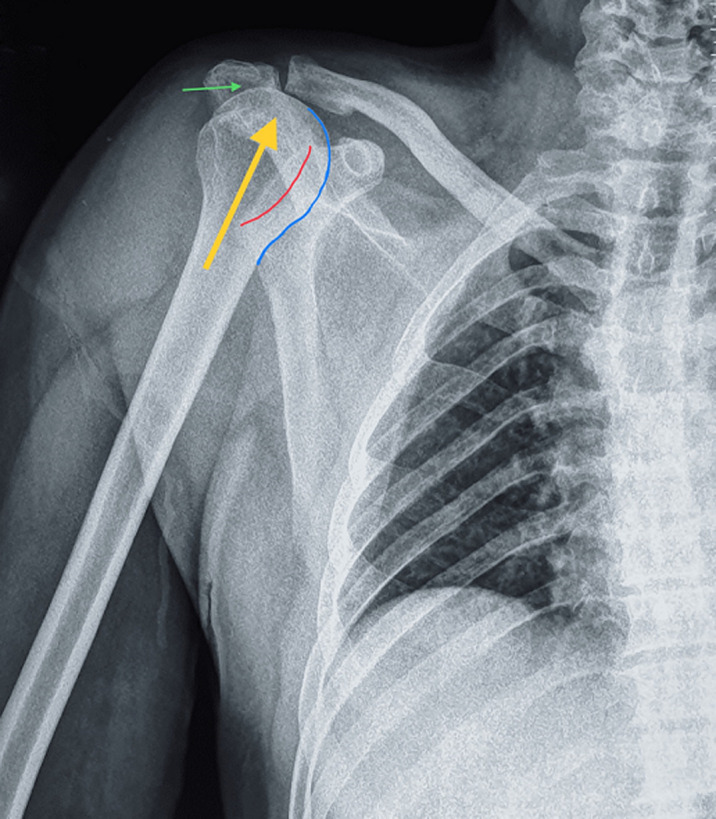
radiographie de face objectivant la luxation antéro-supérieure de l´épaule, (flèche jaune: le déplacement supérieur de la tête humérale; ligne rouge: la limite antérieure de la cavité glénoïde; ligne bleue: contour interne de la tête humérale; flèche verte: comblement de l´espace sous-acromiale par la tête humérale)
